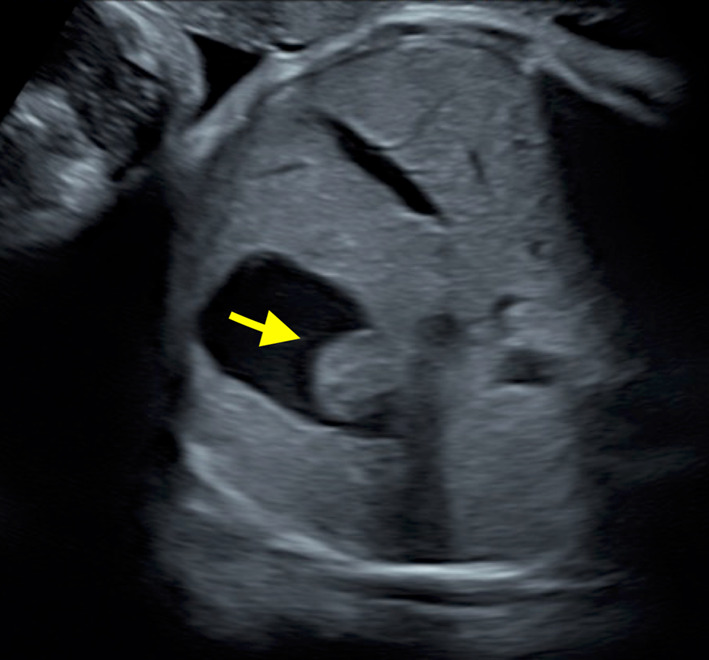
Representative image of a fetal ultrasound study at 21 weeks of gestation. A mass protruding into the lumen of the stomach is observed, demonstrating a mosaic-patterned internal echo (arrow)
